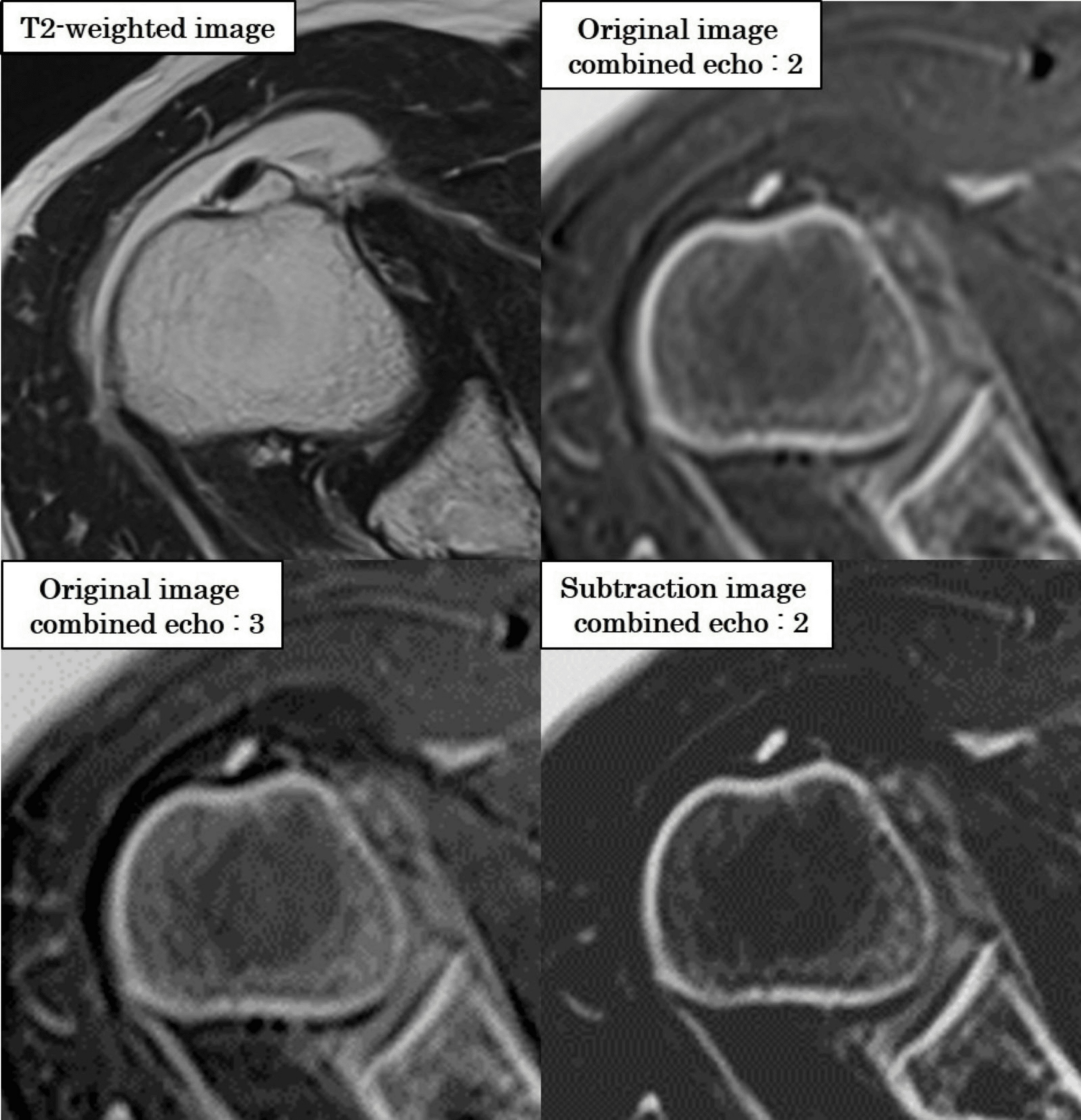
Suppression of water signal with increased number of combined echoes in grayscale-inverted CT-like MRI Representative images show how increasing the number of combined echoes affects signal characteristics. As the number of combined echoes increases (from one to three), the water signal becomes more suppressed due to the inclusion of longer TE components. While this enhances background suppression, it may also reduce contrast between bone and muscle, potentially compromising structural delineation in grayscale-inverted images.
